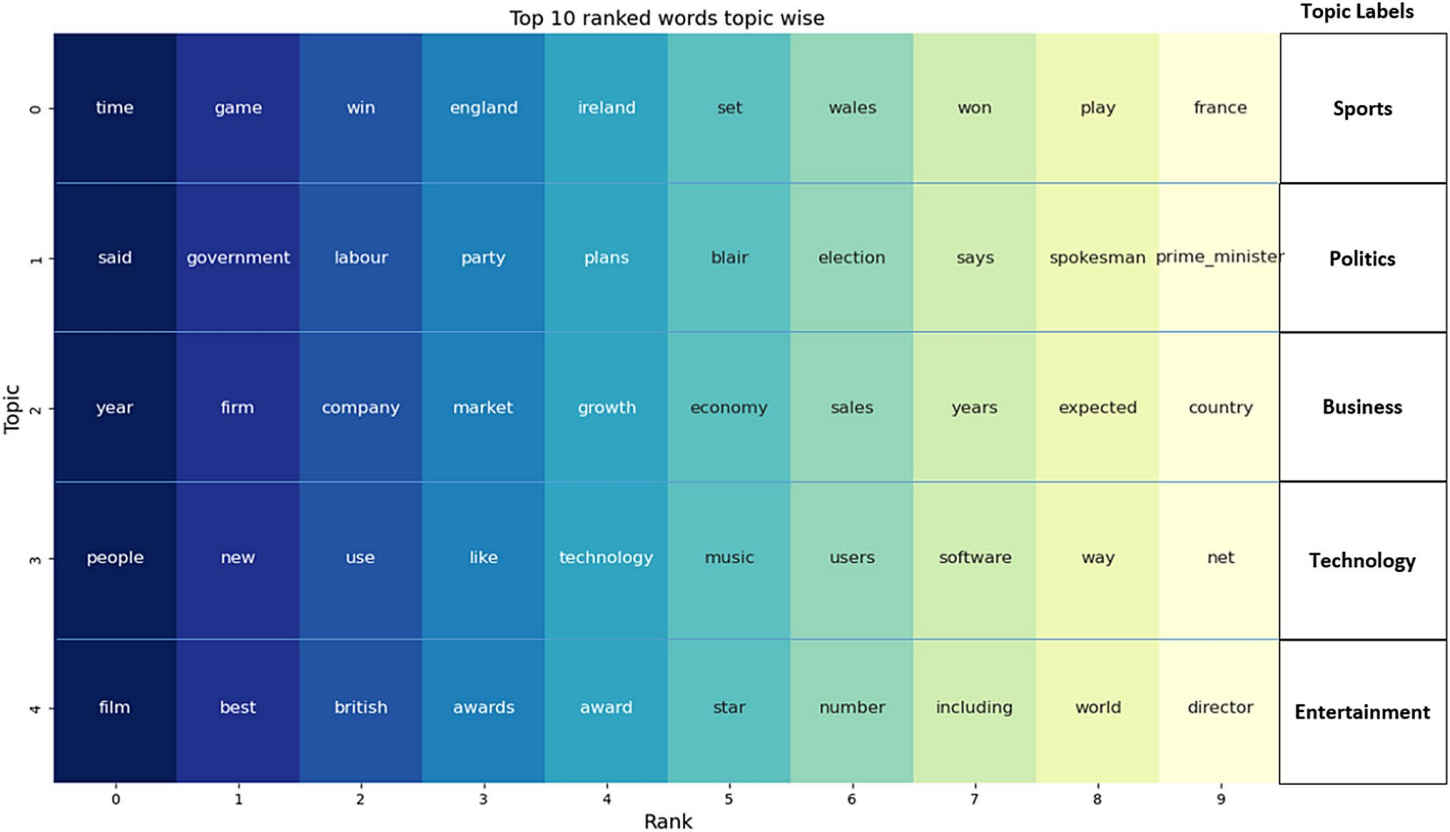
Top 10 words per topic produced by CT on BBC corpus
